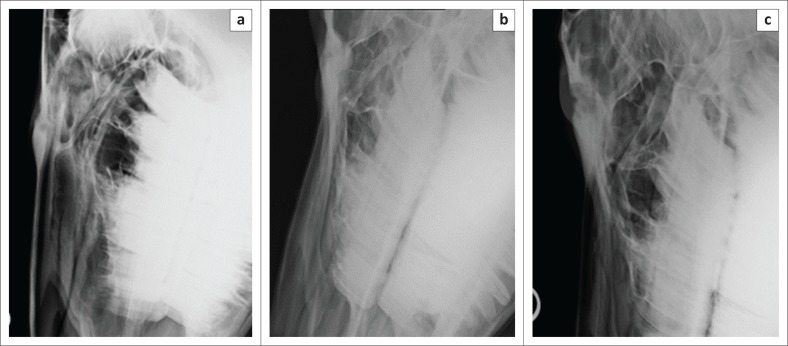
(a) Latero-lateral, (b) LeDRtVO and (c) RtDLeVO radiographic projections of the affected area, demonstrating the smooth solid periosteal reaction associated with an irregular radiolucent fissure line and a poorly marginated oval radiolucency associated with this feature particularly obvious in (c). Also note the depressed appearance of the caudal nasal and rostral frontal bones, especially prominent in (a).
